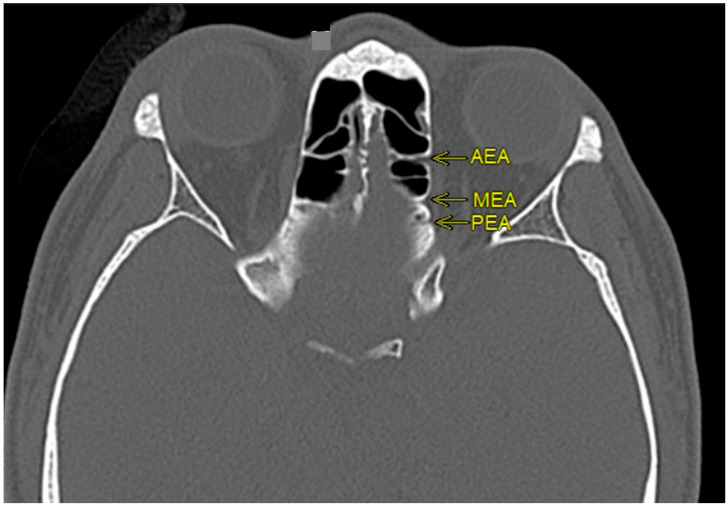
Computed tomography of paranasal sinuses (axial view) of the anterior ethmoidal (AEA), middle ethmoidal (MEA) and posterior ethmoidal arteries (PEA).
